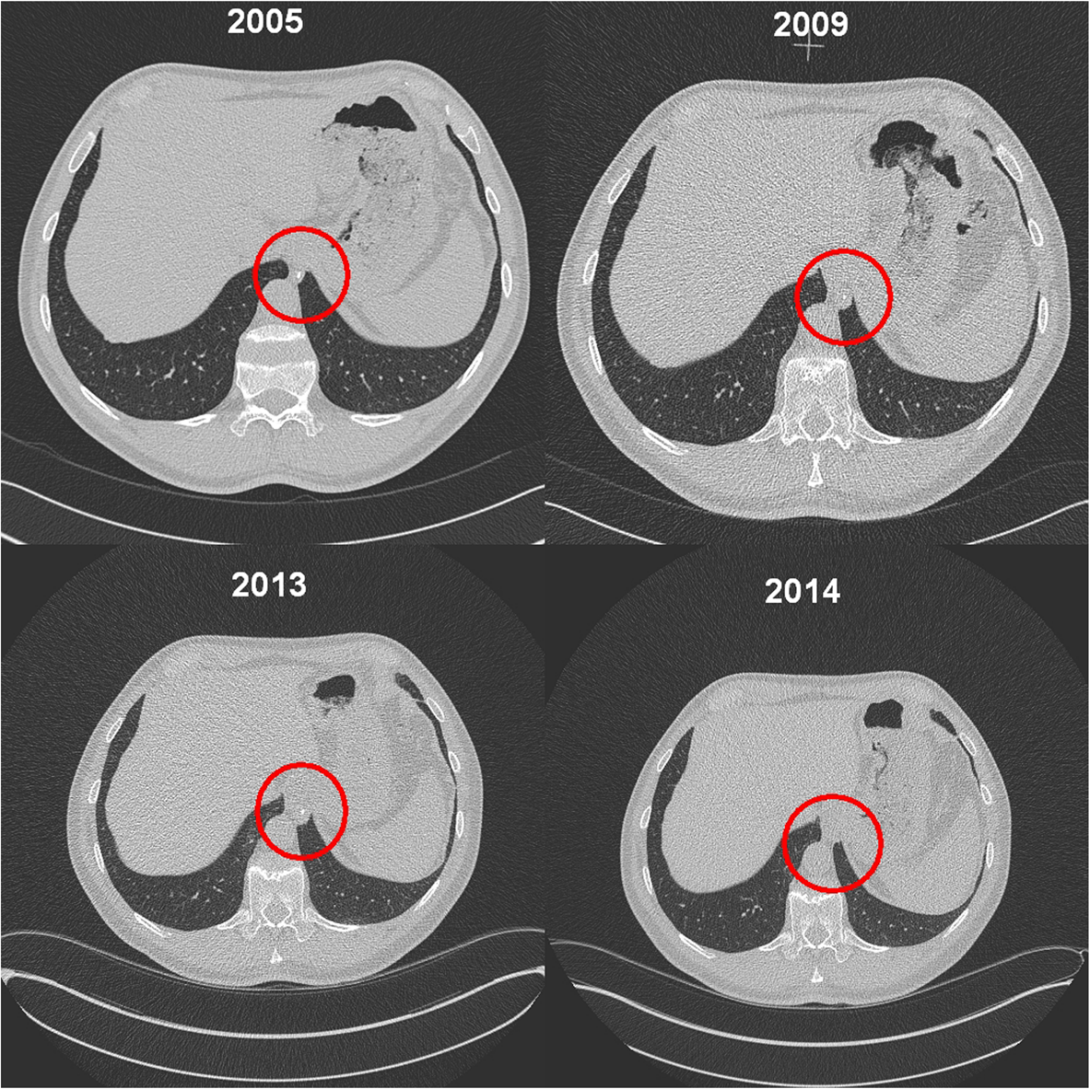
CT-scans taken between 2005 and 2013 showing the growing nodule attached to the mediastinal pleura next to the oesophageal hiatus. The discovery of the presumably same nodule further down the sinus in 2014 triggered further diagnostic interventions
